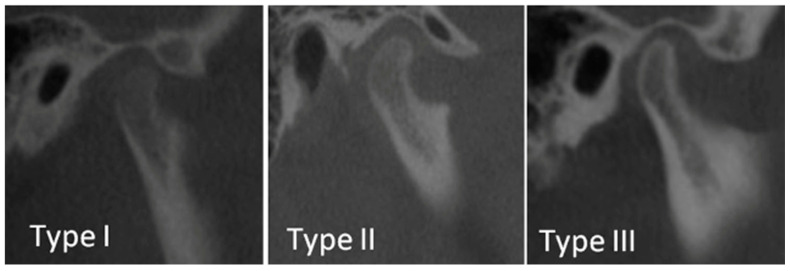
Type 1, 2, and 3 cortication stages of mandibular condyle in CBCT images [14].
